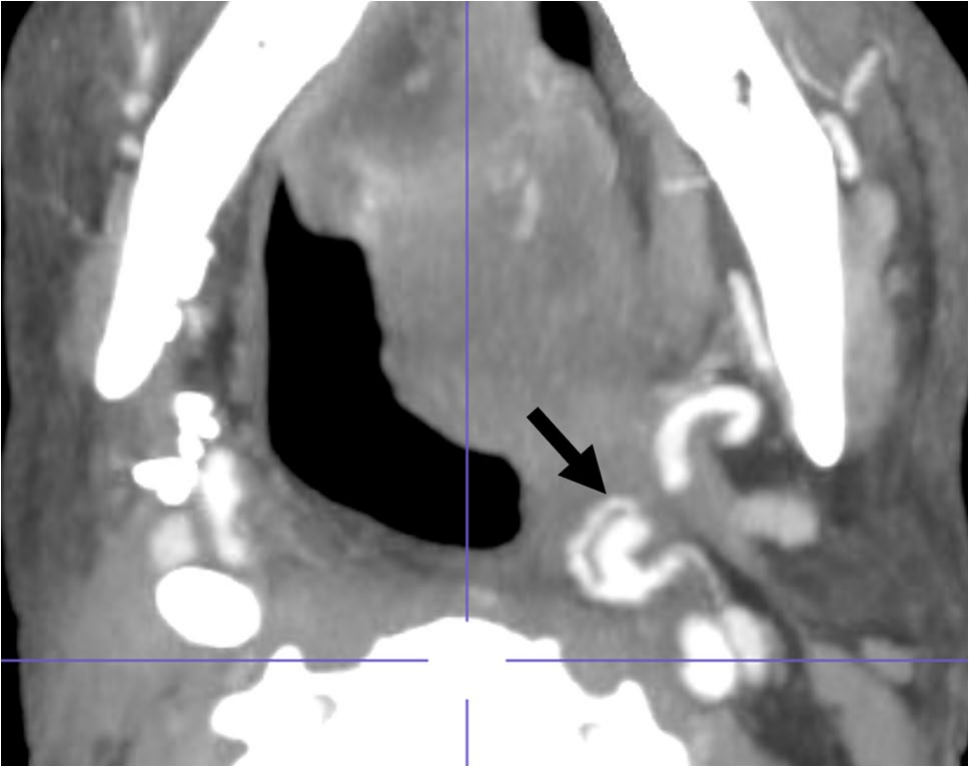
Patient 6. Axial CTA MIP image of the neck at the level of the BOT obtained 10 days following acute presentation demonstrates an irregular appearing left lingual artery (black arrow) extending into the left BOT recurrent tumor bed, likely the source of hemorrhage
